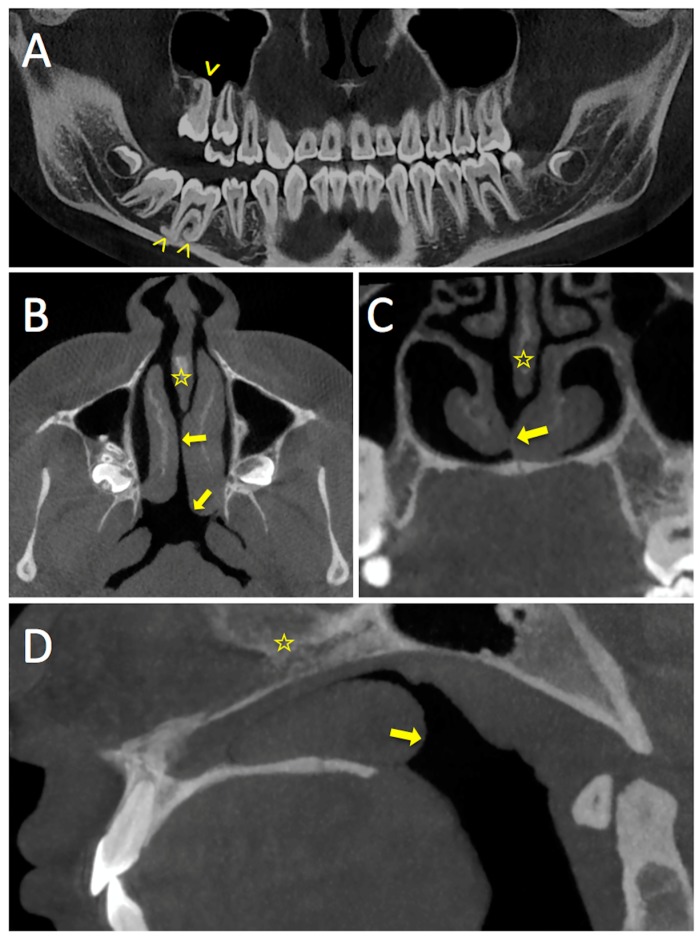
Multiplanar reconstruction of the CBCT acquisition in the curved panoramic-like plane (A) and in the horizontal (B), coronal (C), and sagittal planes (D). Panoramic-like reconstruction shows the hooked aspect of the root apices of teeth 16 and 46 (arrowheads) with primary failure of eruption. The nasal septum is incomplete (yellow stars) with a large defect in its postero-inferior part corresponding to complete absence of the vomer. The bony structures of the middle and lower turbinates appear to be preserved. Nevertheless, the left lower turbinate (arrows) is hypertrophied and protrudes through the septal defect. Note contact between the left and right lower turbinate and posterior protrusion of the left lower turbinate into the nasopharynx.
